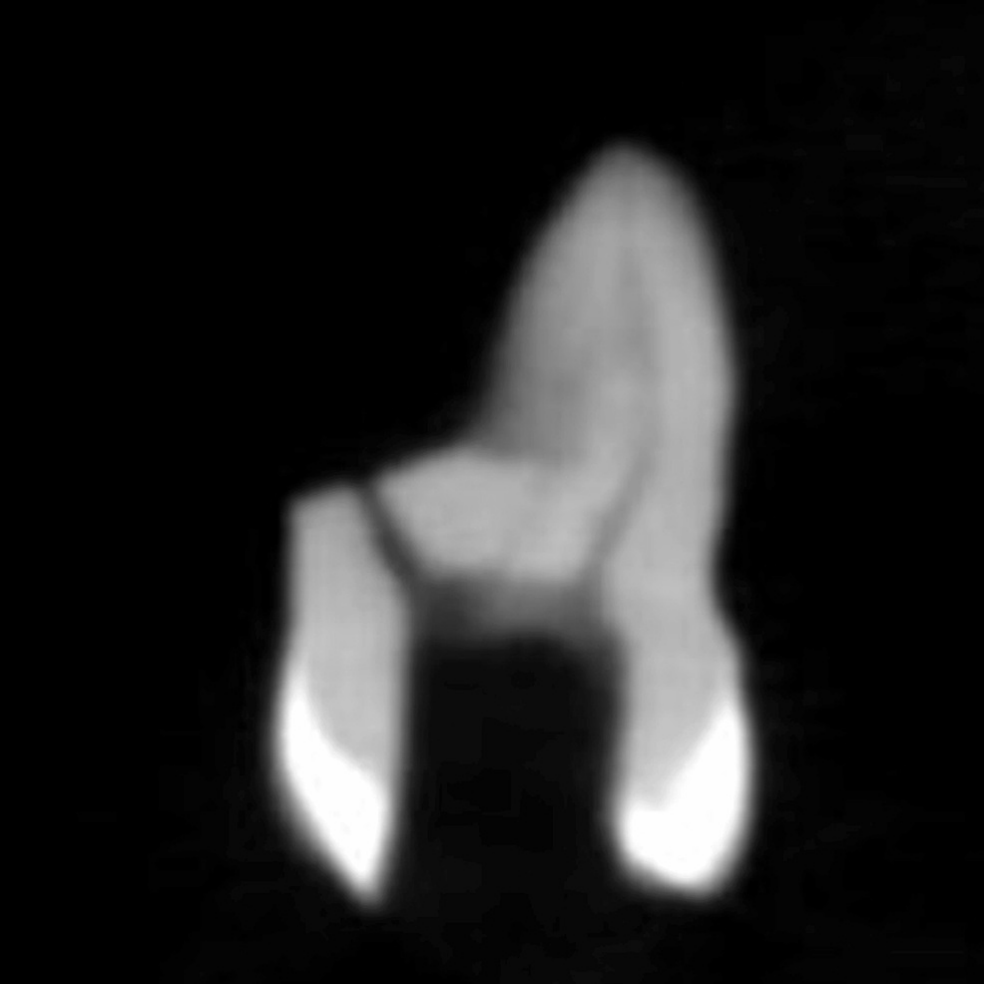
Cone-beam computed tomography (CBCT) scan of the tooth in the study for lateral canal evaluation
